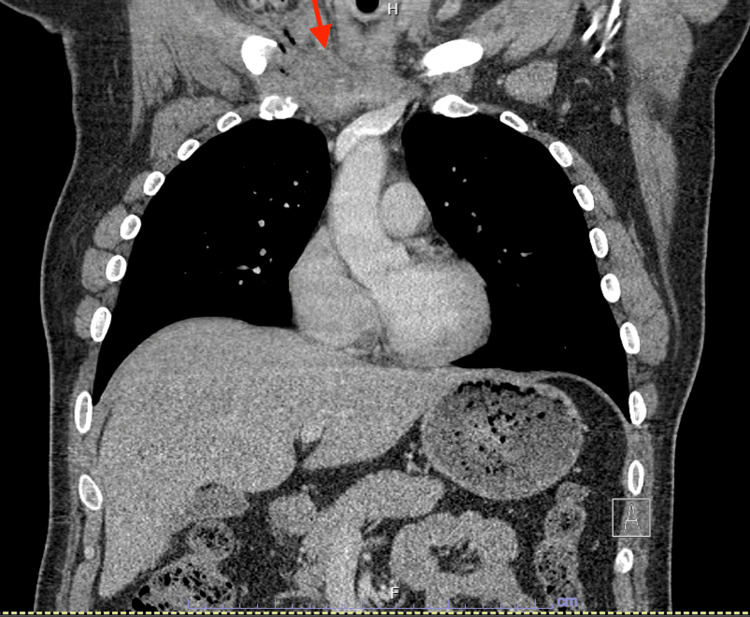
CT scan of the right sternoclavicular joint effusion showing possible extension into the mediastinum (red arrow)
